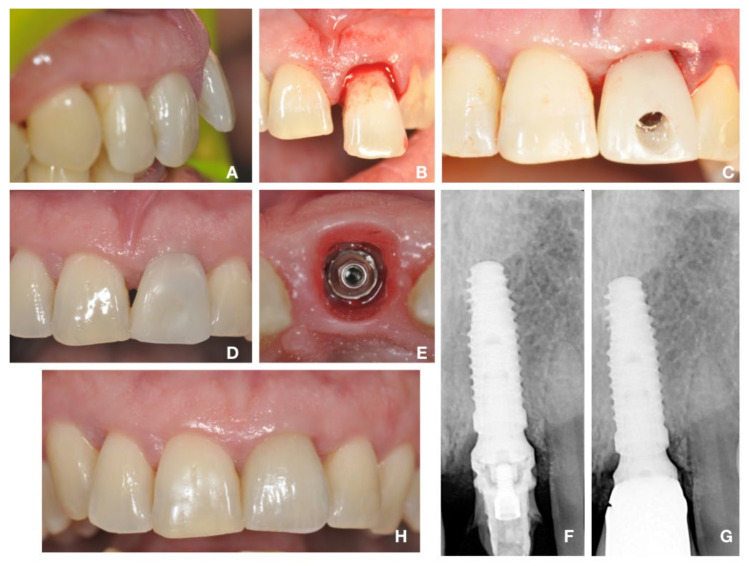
Single restoration in fresh extraction socket, immediately loaded with temporary abutment and prosthesis. (A) Clinical situation before the extraction of tooth 2.1. (B) Extraction of the hopeless tooth 2.1. (C) Implant insertion under the bone crest level, according to the mathematical rule (a low profile MUA was contextually positioned and immediately loaded with a temporary crown). Clinical evaluation after 60 days, showing excellent soft tissue healing around the temporary crown (D) and the implant (E). Radiographic evaluation after 60 days (F) and after 5 years follow-up (G), showing no bone resorption around implant. (H) Clinical evaluation after 5 years follow-up.
